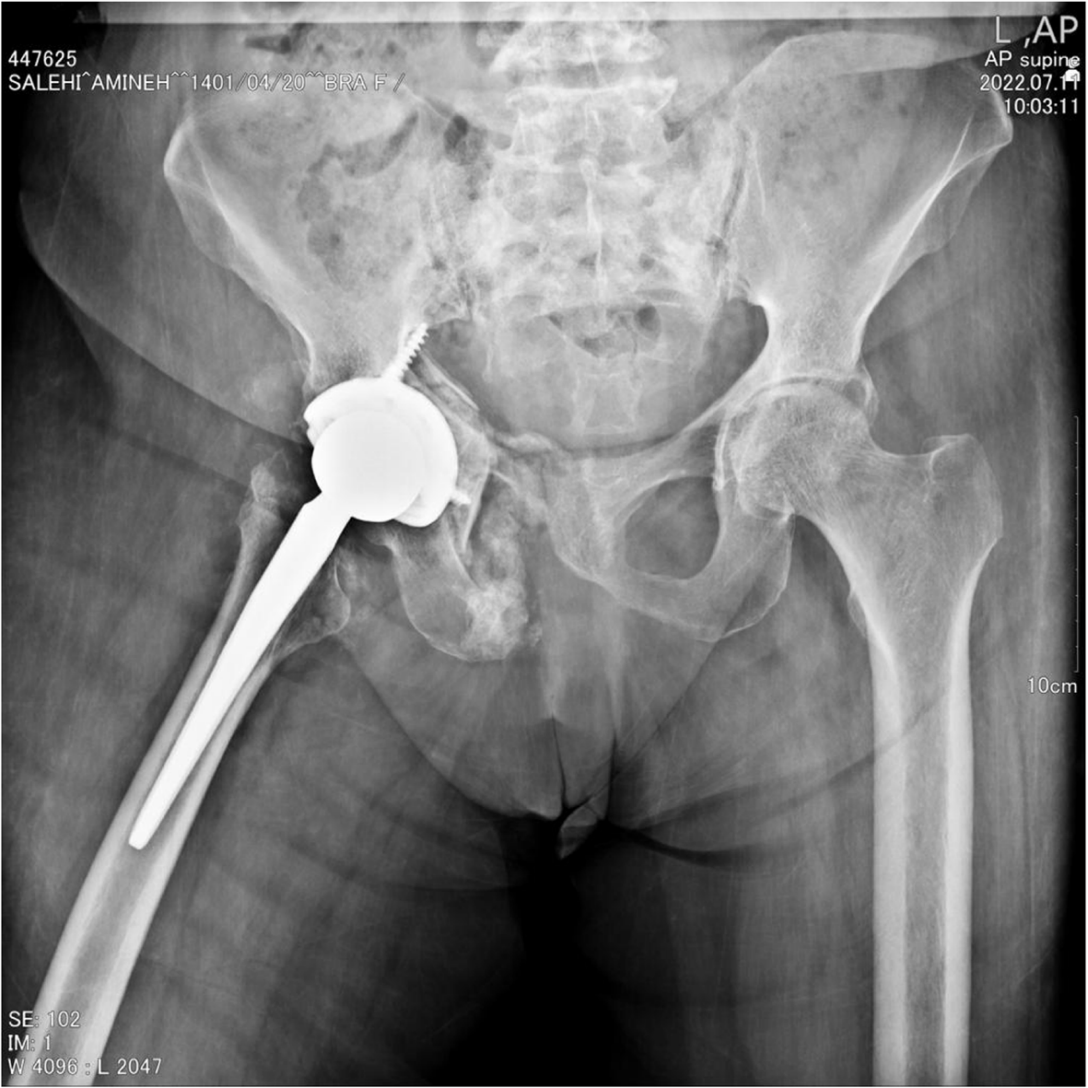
Patient’s post operation radiographs
